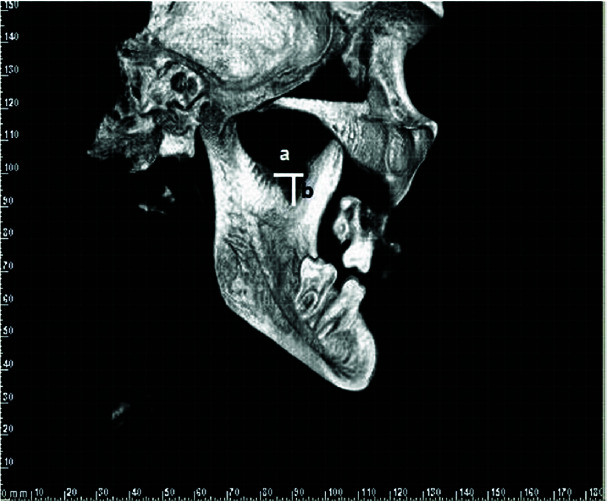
Medial depression of the mandibular ramus (MDMR) measurement: width (a), depth (b) on 3D CBCT images
